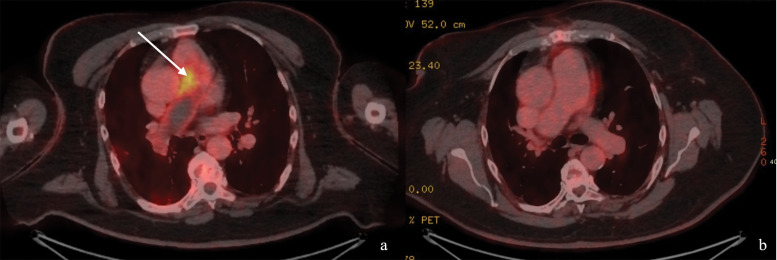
Axial ^18^F-FDG-PET-CT. (a) Three weeks after initial presentation, imaging demonstrating moderate metabolic activity *(white arrow)* in the wall of the pulmonary trunk (GE Medical Systems Discovery MI, PET-WB-QClear600 reconstruction, SUVmax = 6.3). (b) Six-month post-endarterectomy imaging showing no evidence of recurrence.
